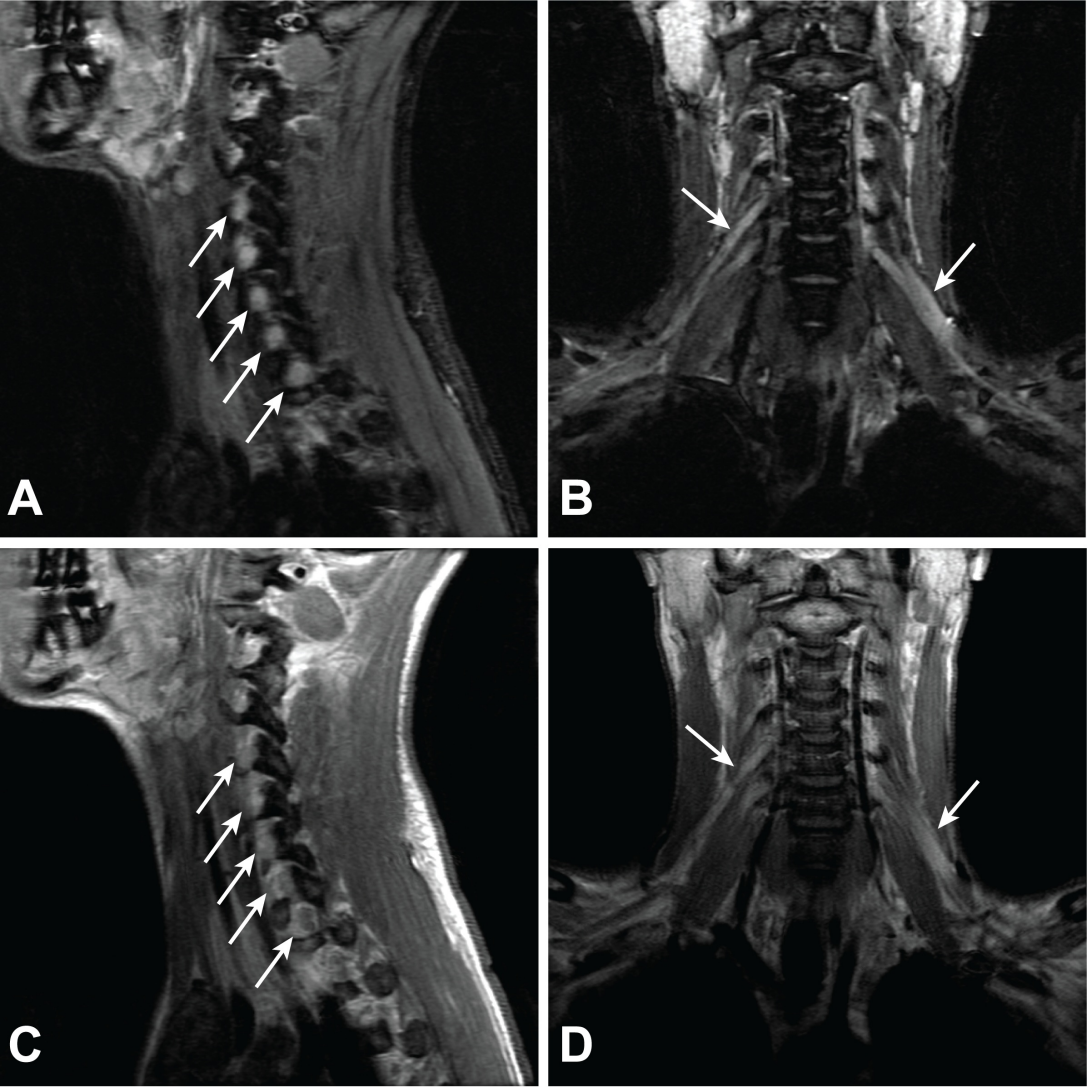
Brachial plexus magnetic resonance imaging demonstrating leukemic infiltration of the bilateral nerve roots. (A, B) Short tau inversion recovery (STIR) images demonstrate nerve root thickening and increased T2-weighted signal abnormality (arrows) in the sagittal (A) and coronal plane (B). (C, D) Post contrast images show diffuse enhancement within thickened nerve roots of the brachial plexus in the sagittal (C) and coronal plane (D).
